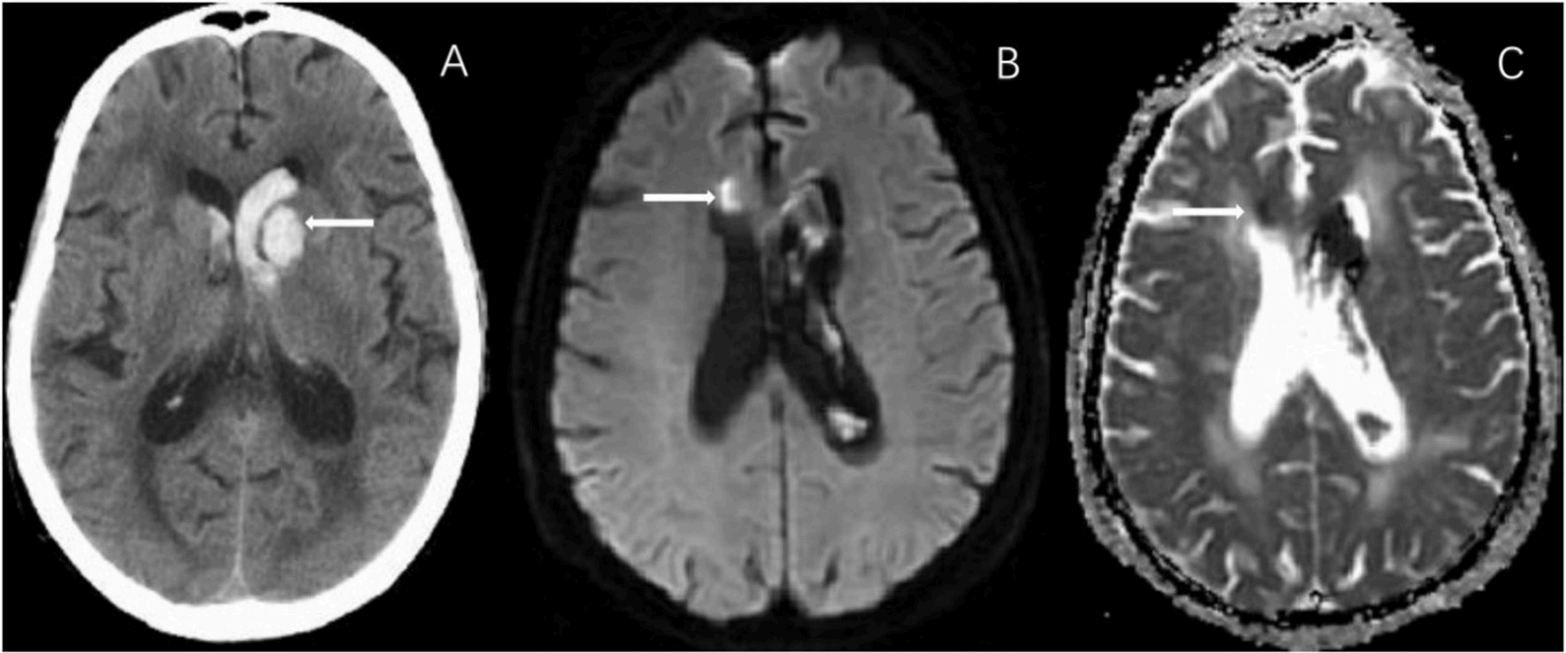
In a 75-year-old female with left basal ganglia hemorrhage (A), diffusion weighted imaging (DWI) shows a small remote ischemic lesion on right frontal angle (B), with corresponding low signal intensity in apparent diffusion coefficient (ADC) map (C).
